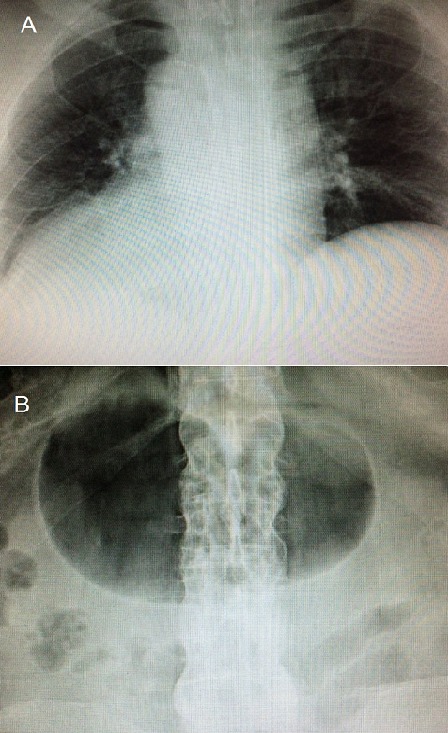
(A) chest X-ray showing right-sided aortic arch and cardiac apex pointing to the right; (B) abdomen X-ray demonstrating a dilated right-sided stomach bubble
